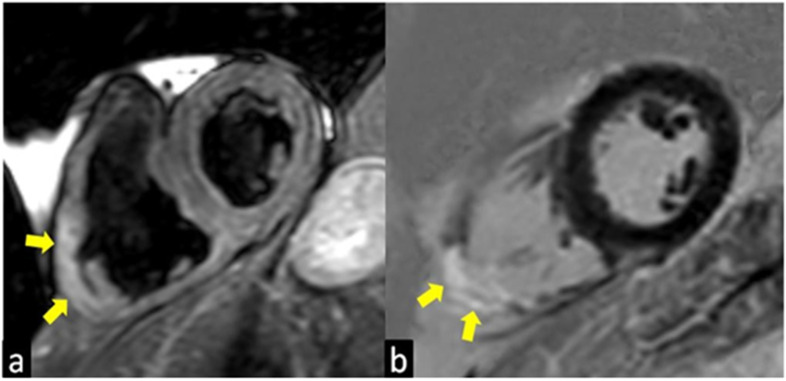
T2-weighted (a) and Late Gadolinium Enhancement (LGE; b) images show a case of atypical presentation of the acute phase of Cardiac Sarcoidosis, characterized by the presence of edema (yellow arrow in a) and LGE (yellow arrow in b) of the inferolateral wall of the right ventricle with the transmural distribution.
